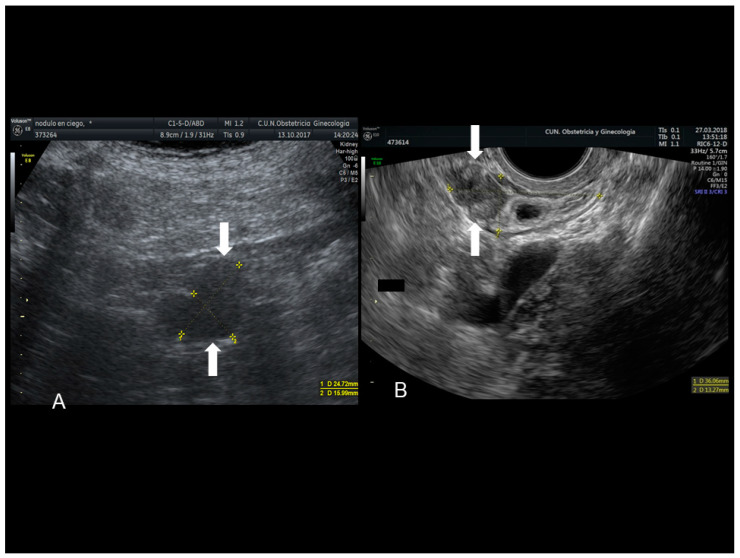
Sonographic features of an endometriotic nodule of cecum (see white arrow) (A) and appendix (see white arrow) (B).
